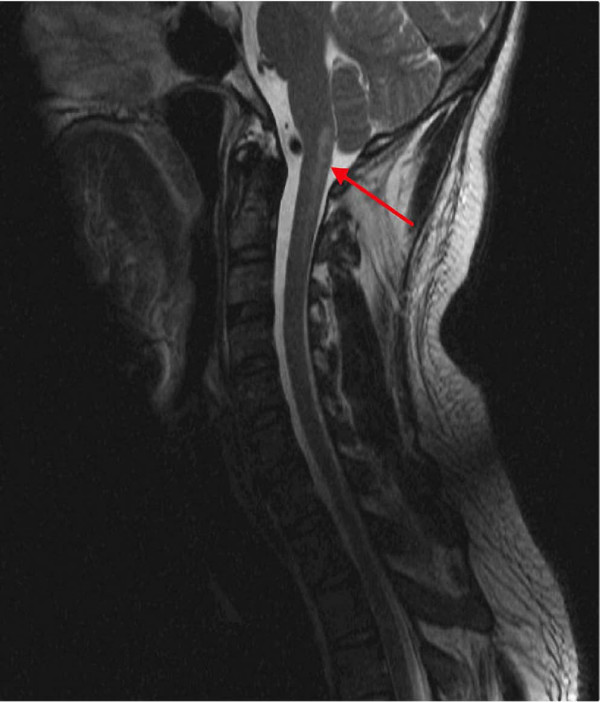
Sagittal T2 restore magnetic resonance image demonstrating a posterior hyperintense lesion (red arrow) at the cranio-cervical junction involving the caudal medulla and the upper cervical cord.
